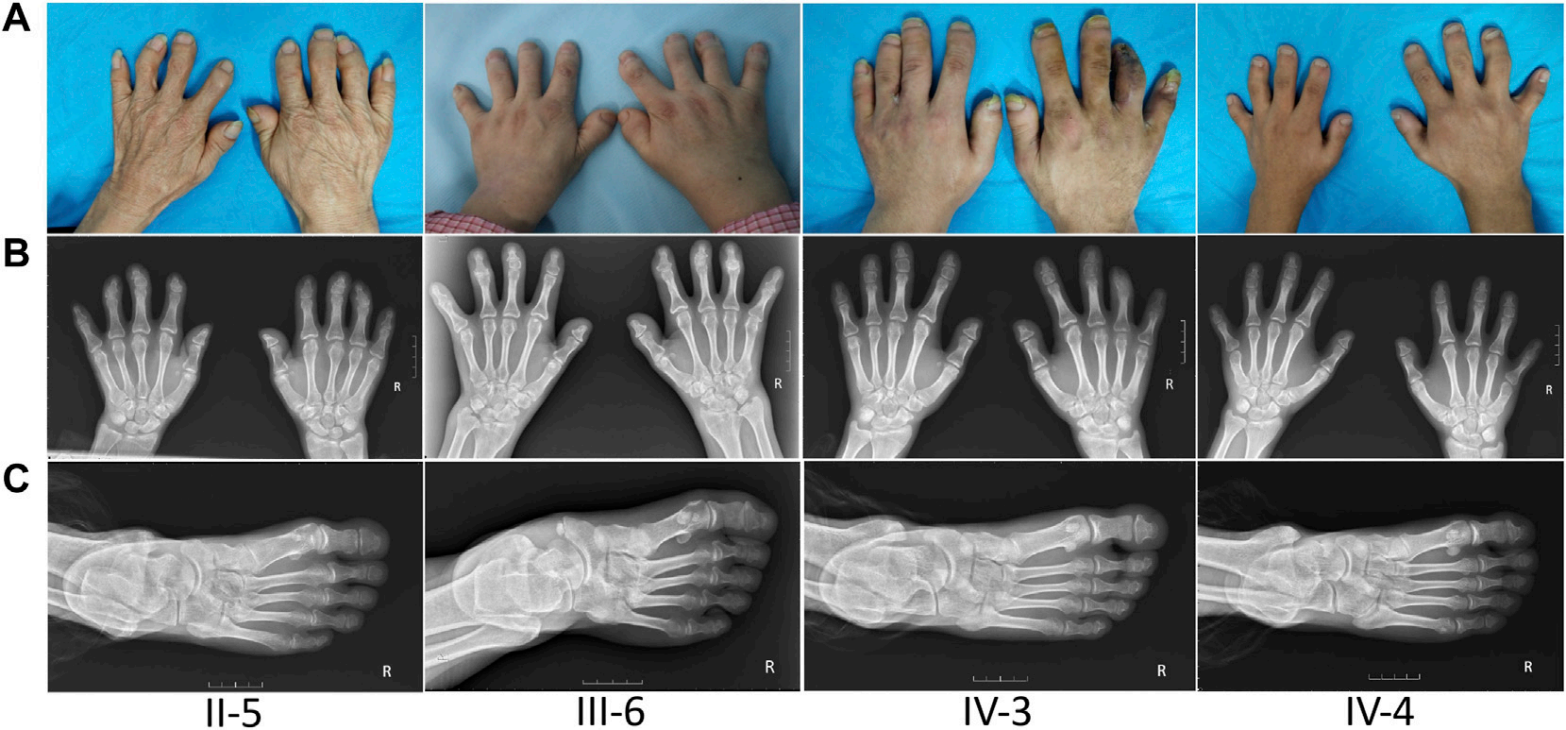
Appearance and radiological findings of other family members with BDA1. (A) Shortened fingers and radial deviation of other affected individuals in this family. (B) Radiographic images of other patients’ hands: absence of middle phalanges of the second, fourth, and/or fifth fingers (II-5, II-6, IV-3, IV-4) in both hands, and the middle phalanges of the second finger of the right hand were fused to the distal phalanges (IV-4). (C) Radiographic images of other patients’ right foot: abnormally shortened toes and the absence of phalanges.
